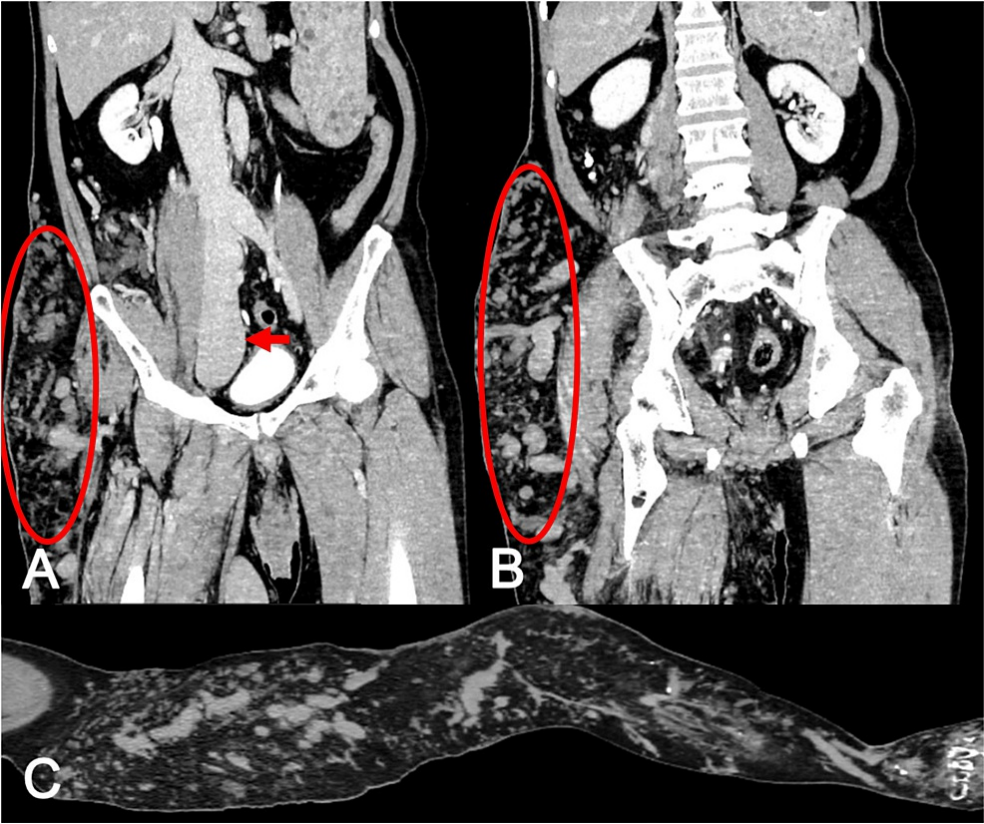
Contrast-enhanced CT. (A, B: Axial view; C: Sagittal view). Abnormally developed varicose veins can be observed mainly in the right thigh (oval). A dilated inferior vena cava can also be observed in panel A (arrow). Panel C shows a sagittal image of the right lower extremity at the level of the lateral border of the bone. In panel C, the right lower extremity at the level of the lateral border of the bone shows well-developed varicose veins, mainly in the thigh, the popliteal fossa, and the lower leg.
